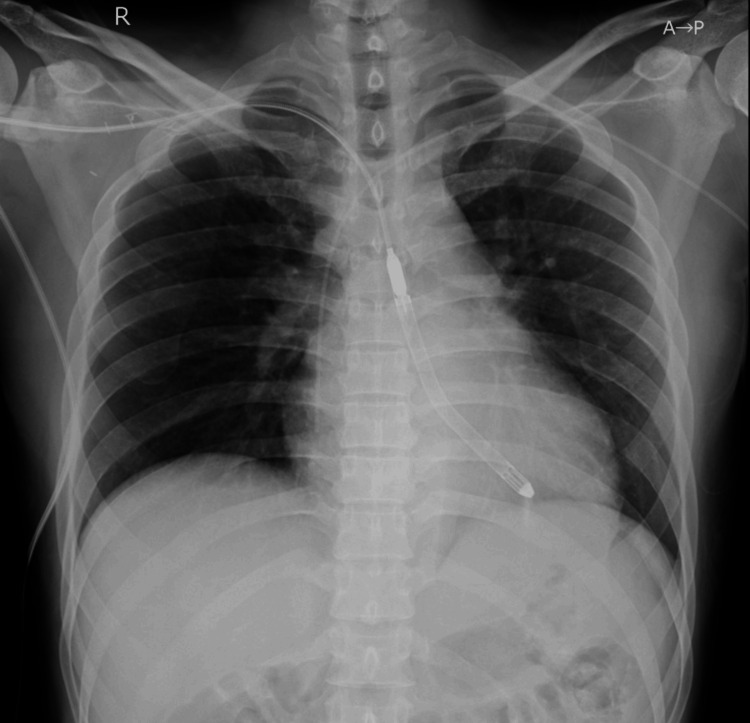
Chest X-ray His chest X-ray showed that the Impella 5.5* was inserted through the right axillary approach. No congestion was observed. *Abiomed, Inc., Danvers, Massachusetts, United States
